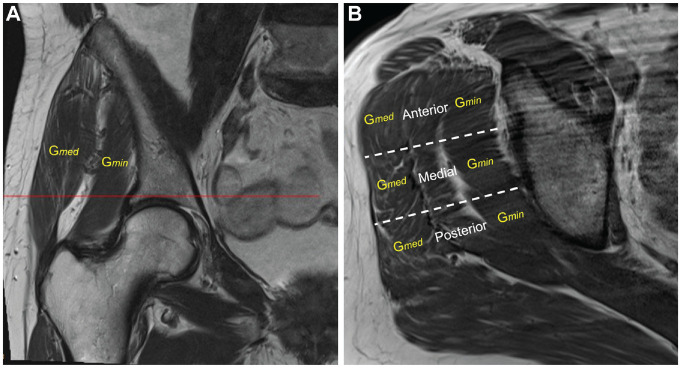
(A) Coronal T1-weighted magnetic resonance image of a right hip
